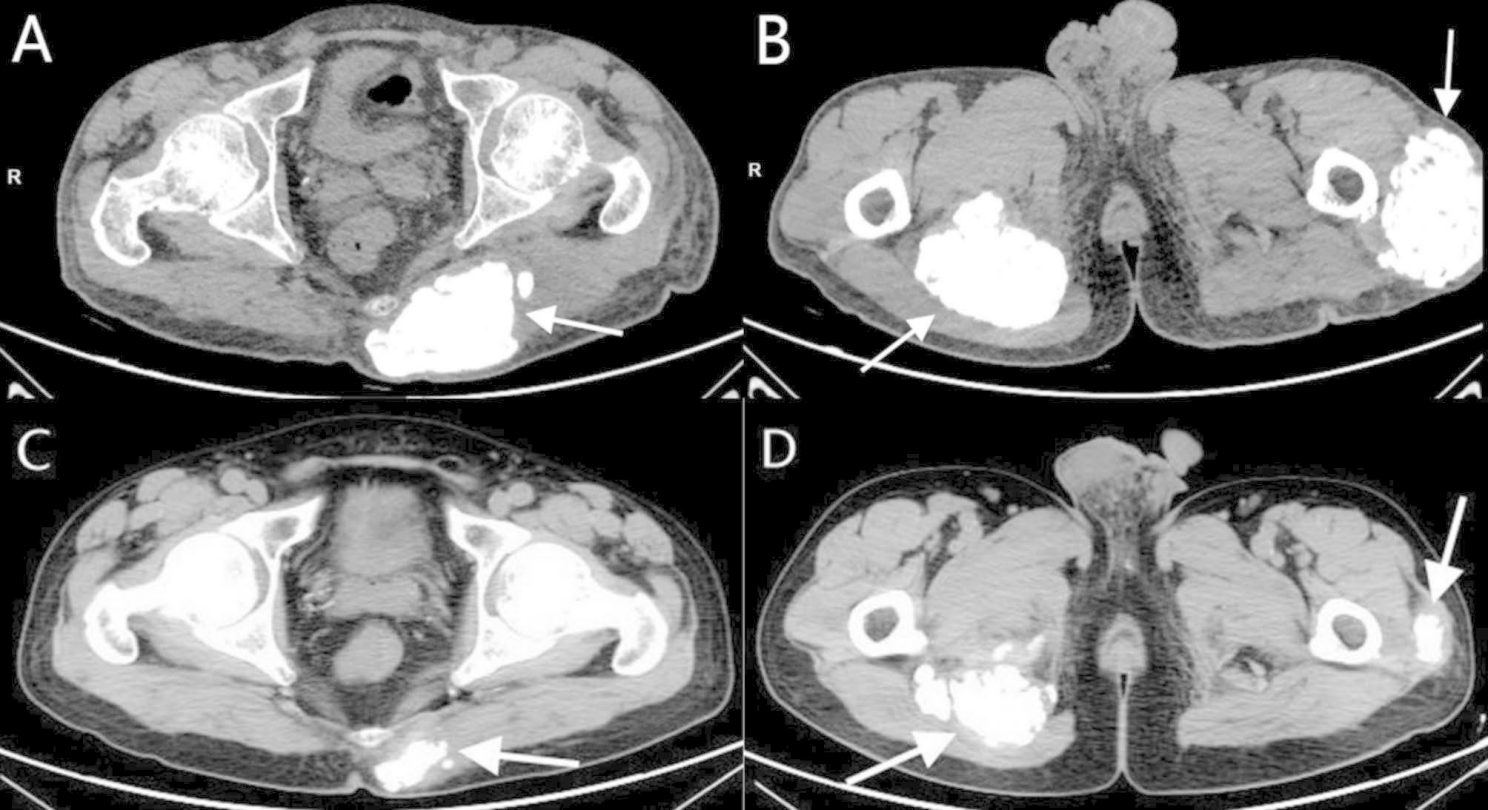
CT scan of the pelvis before (A, B) and after parathyroidectomy (C, D)in case 1
